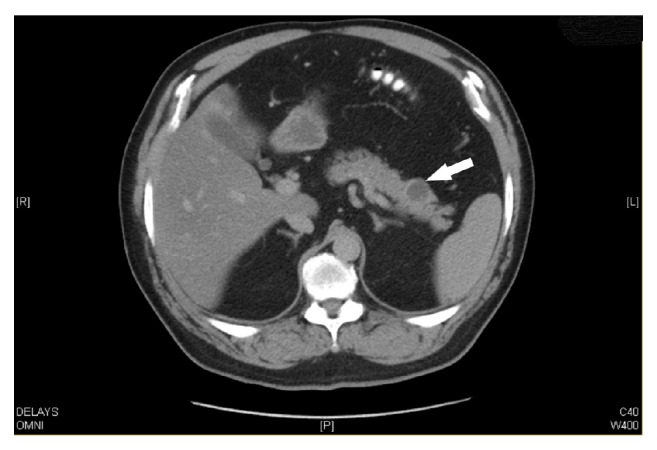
CT abdomen pelvis performed after EUS-FNA aspiration showing persistence of the cyst (arrow).
